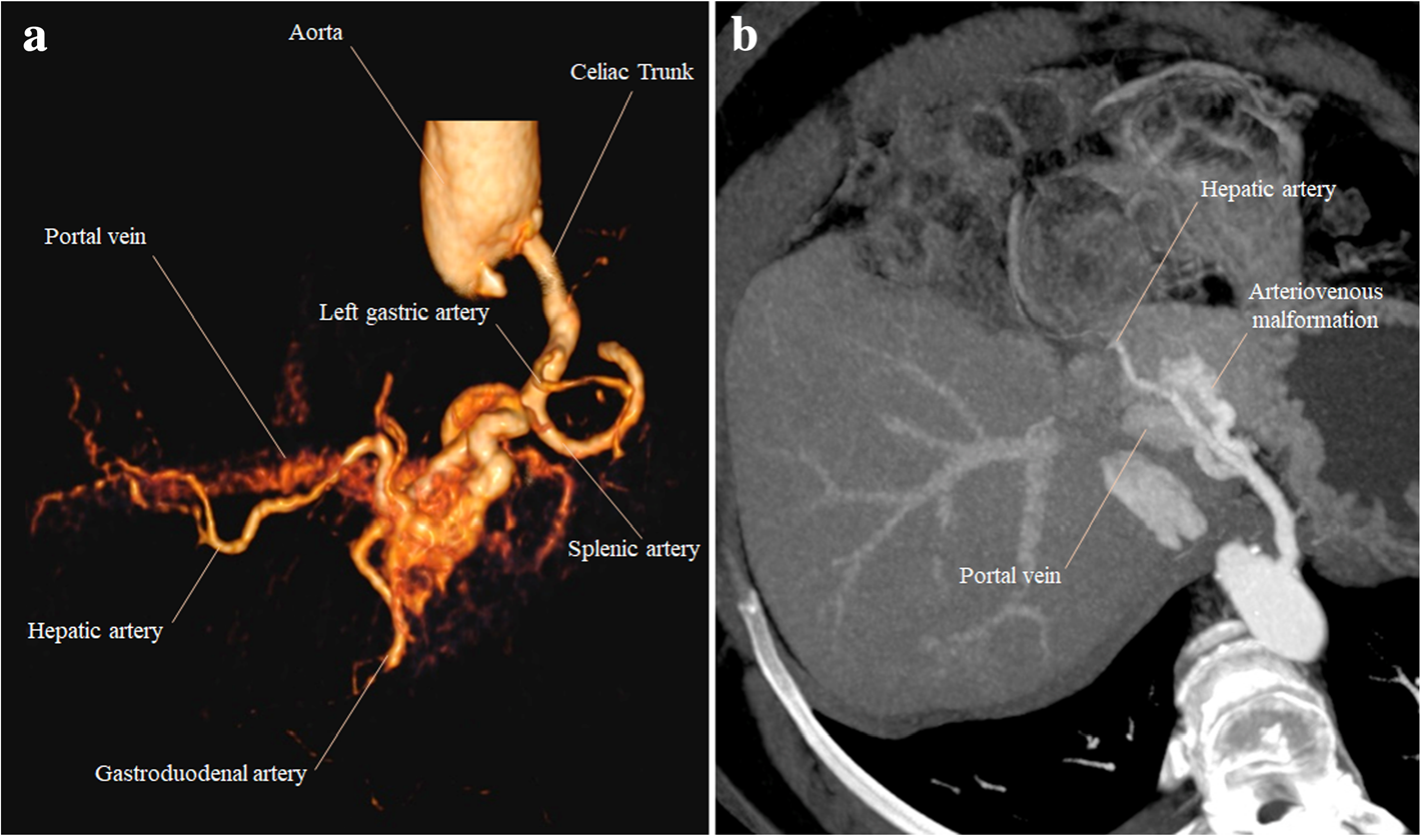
Congenital extrahepatic arterioportal fistula. a VRT and b MIP images from dominant arterial phase CT. a Best depict an early enhancement of the PV in this phase, a sign of arterioportal shunt. b Here, we can precisely individualize the arteriovenous malformation, as an aberrant tortuous hepatic artery branch connecting with the left PV, with an attenuation similar to the hepatic artery, filling retrogradely the PV
